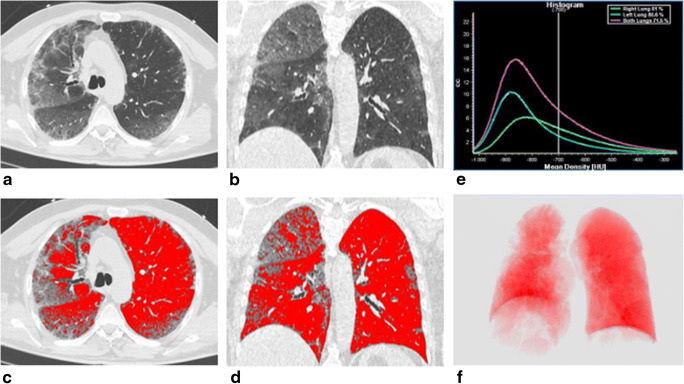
A 63-year-old man with SARS-CoV-2-related pneumonia. CT images in axial (a) and coronal (b) planes, showing the presence of bilateral and peripheral ground-glass opacities associated with septa thickening and crazy-paving pattern, bronchiectasis, and band thickening. Final CT score: 18. Post-processed CT images in axial (c), coronal (d) sections, and 3D reconstruction displaying the ventilated lung volume, characterized by attenuation value lower than − 700 HU (f). The density histogram (e) summarizes the distribution of lung parenchymal density of total lung volume (purple line), left lung volume (green line), and right lung volume (blue line)
